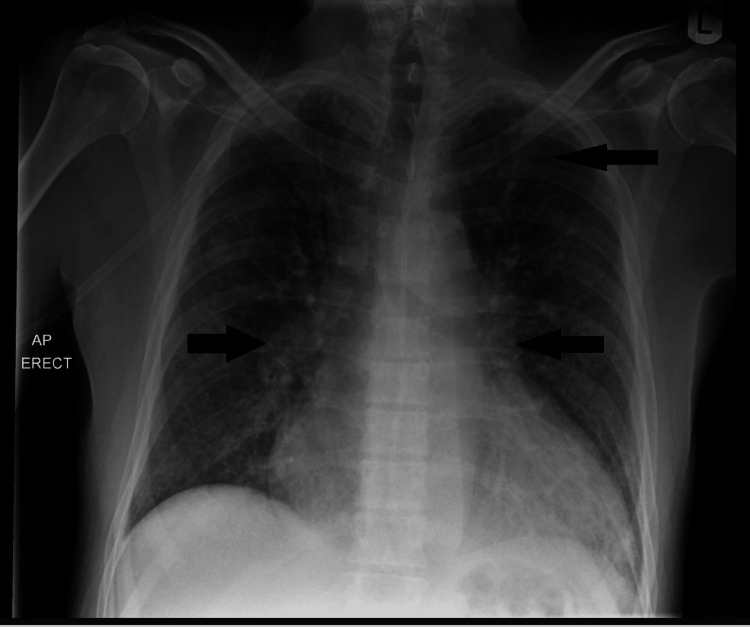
Chest radiograph demonstrating pulmonary oedema Anteroposterior chest radiograph demonstrating pulmonary vascular congestion and bilateral perihilar pulmonary oedema highlighted by the arrows.
